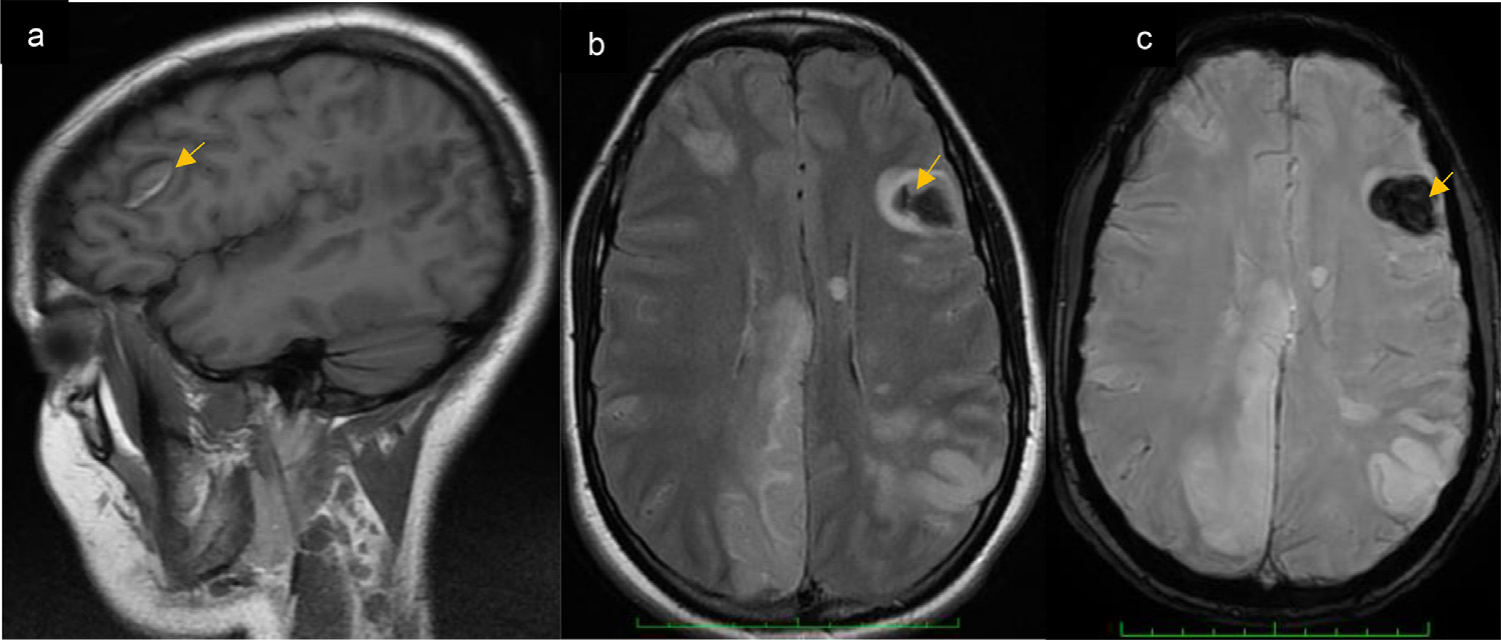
(A) Sagittal T1WI, (B) Axial FLAIR MR, and (C) T2*WI showing a left frontal lobe hemorrhage.
